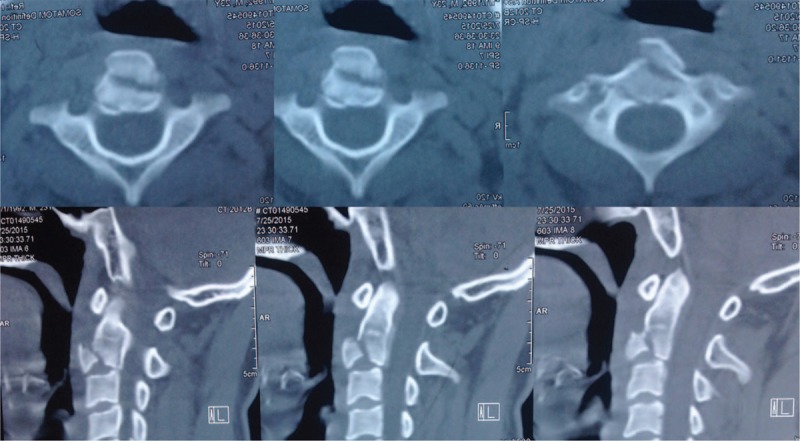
Preoperative three-dimensional reconstruction CT confirmed huge tear drop fracture of anterior-inferior corner of the axis, and the discontinuity of the cortex thereof. CT = computed tomography.
